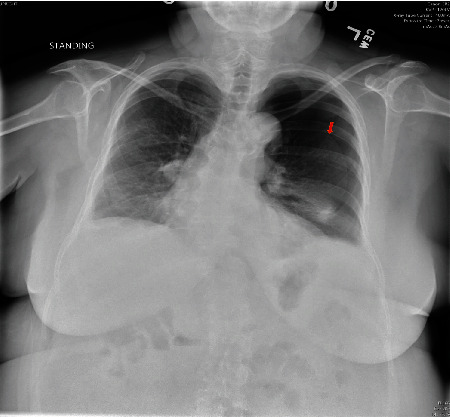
Chest X-ray showing large left-sided pneumothorax and lung collapse (depicted by red arrow).
